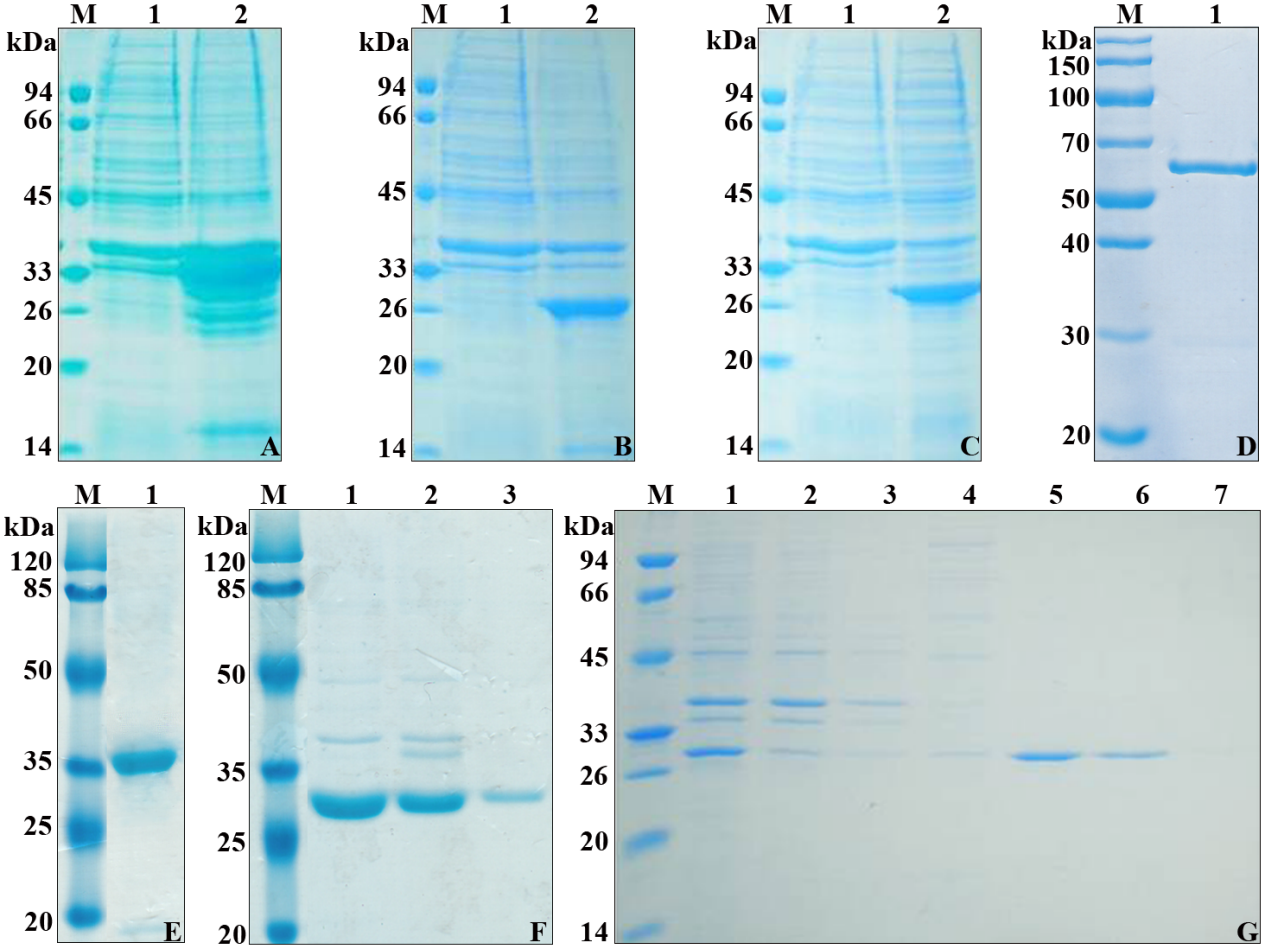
Expression and purification of IE648 and individual domains of Cry1Ie in *E*. *coli* BL21/DE3. A, B and C: Induced expression of domains I, II and III, respectively. Lane M, Protein molecular marker. Lane 1 and 2, supernatant and pellet of bacteria after ultrasonication. Bands of the expected size of 34.6 kDa for domain I, 27.3 kDa for domain II and 27.9 kDa for domain III were present in the pellet fraction. D: SDS-PAGE analysis of purified IE648. Lane M, Protein molecular marker. Lane 1, Purified IE648. E: SDS-PAGE analysis of purified domain I. Lane M, Protein molecular marker. Lane 1, Purified domain I. F: Purification of domain II by Ni-NTA affinity chromatography. Lane M, Protein molecular marker. Lane 1, Inclusion bodies solubilized by using solubilization solutions of Inclusion Body Solubilization and Refolding Kit. Lane 2, Proteins passed through Ni-NTA agarose. Lane 3, Proteins washed by Na_2_CO_3_ buffer (pH 10.2). G: Analysis of purification of domain III by Ni-NTA affinity chromatography. Lane M, Protein molecular marker. Lane 1, Inclusion bodies solubilized by Na_2_CO_3_ (pH 10.2). Lane 2, Proteins passed through Ni-NTA agarose. Lane 3, Proteins washed by Na_2_CO_3_ buffer (pH 10.2). Lane 4~7, Proteins eluted by 20 mM, 50 mM, 100 mM and 150 mM imidazole, respectively.
